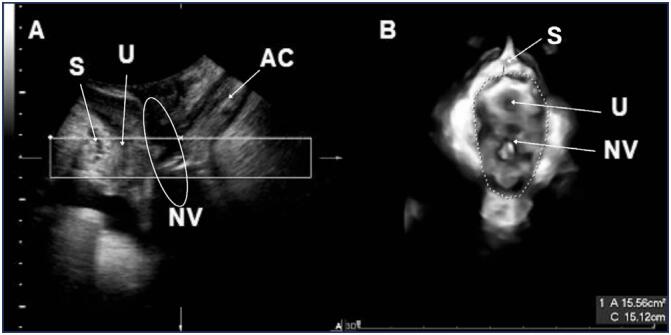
Transperineal ultrasound image to measure the genital hiatus area during the Valsalva maneuver in transgender women submitted to gender-affirming surgery. Pernambuco, Brazil, 2017. A. Image in the mid-sagittal plane to identify pubis symphysis (S), urethra (U), neovagina (NV) and anal canal (AC). B. Axial view of the genital hiatus area during the Valsalva maneuver to identify urethra (U) and neovagina (NV)
